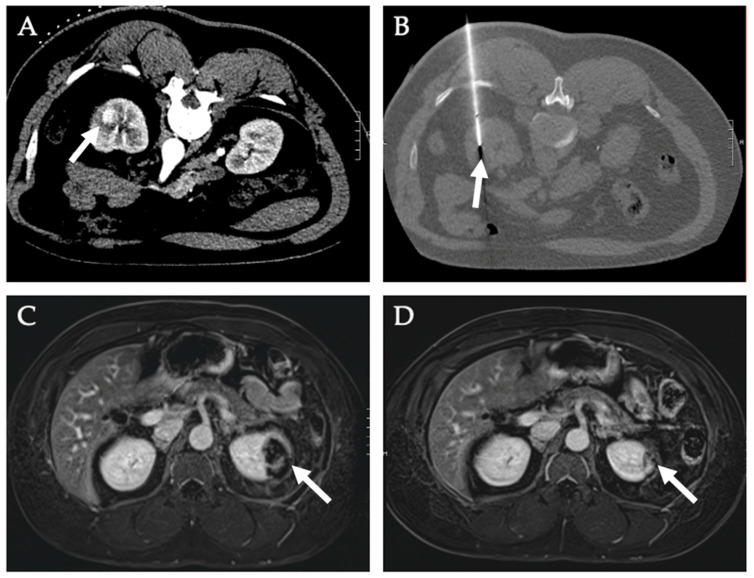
Cryoablation of left renal mass. A 57-year-old male with hematuria and left flank pain was found to have a left renal enhancing lesion (white arrow) on MRI images (A). (B) CT-guided percutaneous core needle biopsy found the 2.0 × 1.8 cm right renal lesion to be medullary fibroma while the 2.1 × 2.2 cm left renal lesion was International Society of Urologic Pathologists (ISUP)/World Health Organization (WHO) grade 2 RCC. The patient underwent cryoablation of the left mass. (C) One-month follow-up MRI showed complete tumor response. (D) Six-month follow-up MRI showed lesion shrinkage without residual tumor.
